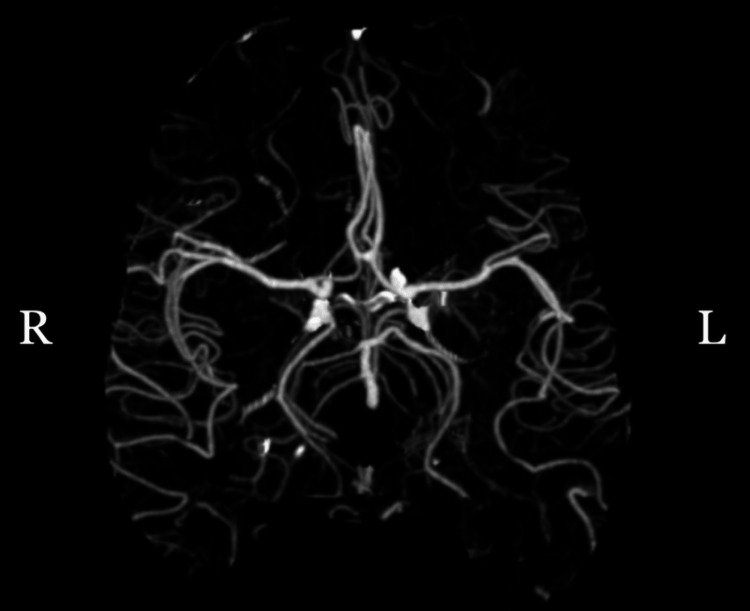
No vascular abnormality is seen on the brain MRI
